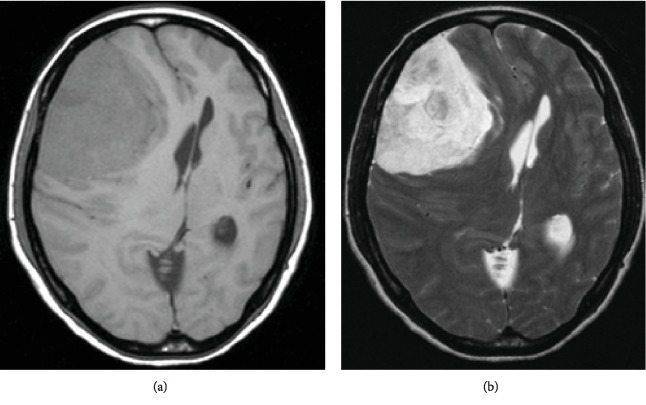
(a) Head magnetic resonance imaging (MRI) revealed a mass presenting homogeneous hypointense signals on T1 images. (b) At the same site, hyperintense signals with a clear border on T2 images.
